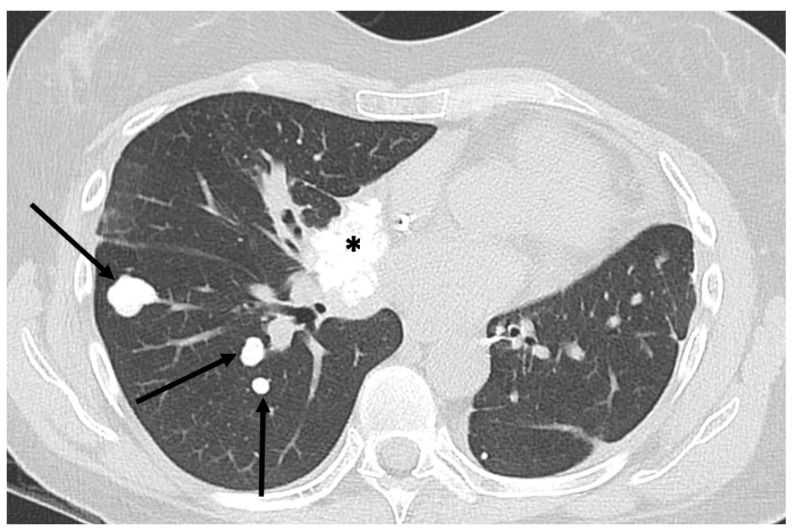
Mineralized bone metastases from extraskeletal osteosarcoma. Axial CT scan (lung kernel) in 54-year-old woman with calcified pulmonary (arrows) and mediastinal (asterisk) metastases.
